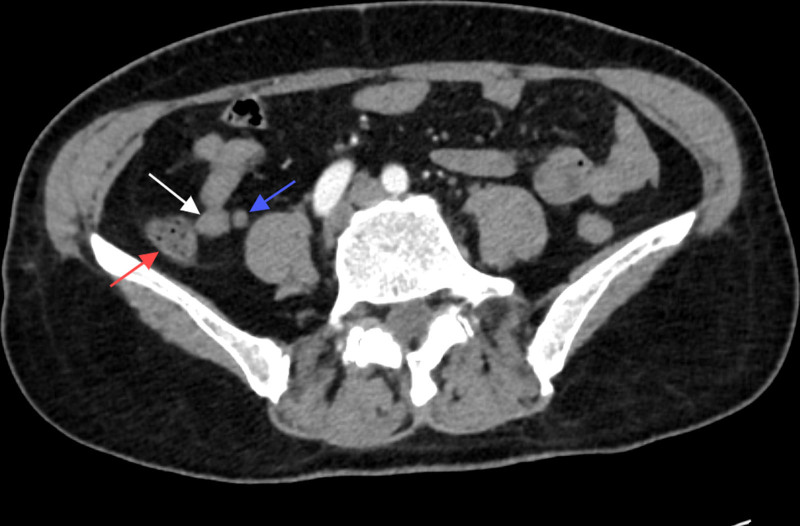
Computed tomography image (transverse section) shows a normal appendix (blue arrow), cecum (red arrow), and terminal ileum (white arrow).
